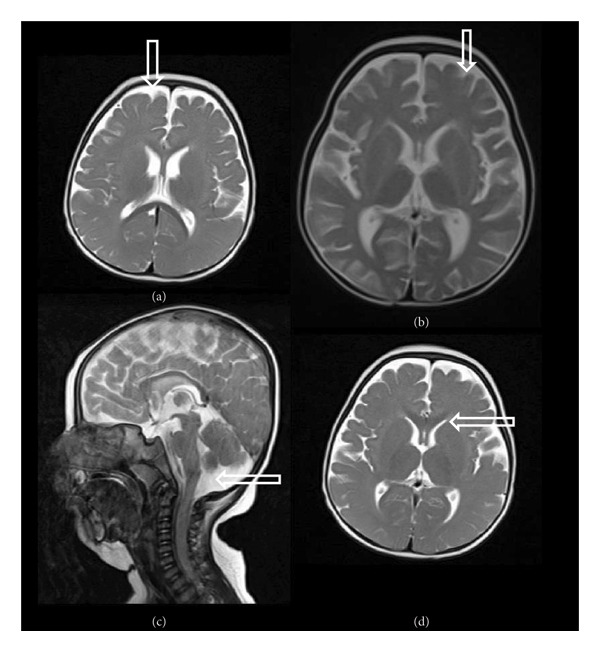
MRI study of proband. (a, b) Baseline magnetic resonance imaging examination performed at 3 years in subject V‐4 revealed brain atrophy with primary Sylvian fissure secondary to frontotemporal atrophy. (c) Increased size of retrocerebellar cisterna. (d) Diffuse symmetrical increase of signal intensity on periventricular white matter and subcortical white matter and corpus callosum thinning.
